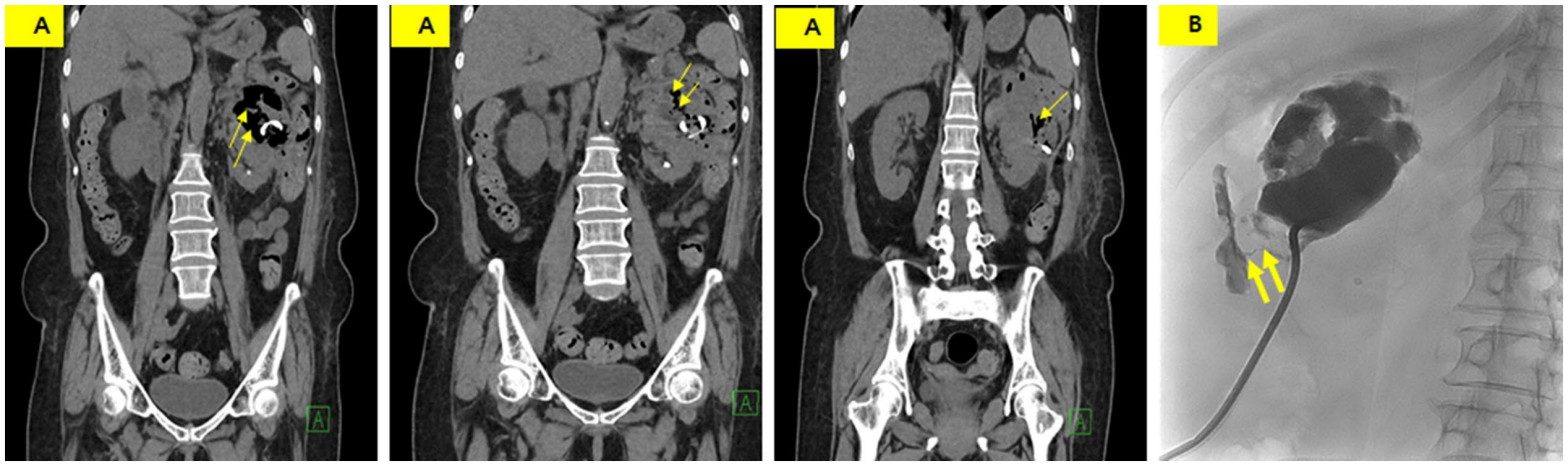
(A) Non-enhanced CT showing a slightly decreased abscess size with new intralesional air (thin arrow) and an indistinct boundary between the perinephric abscess and descending colon. (B) Tubogram demonstrating an abscess pocket measuring 7 cm in length, with a fistula tract (thick arrow) showing contrast entering the descending colon.
